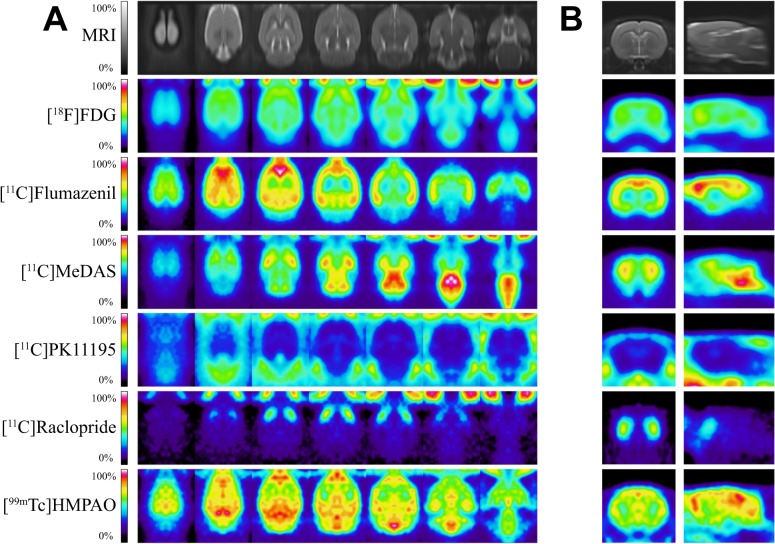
Tracer-specific PET and SPECT templates. (A) Different horizontal brain sections, and (B) sagittal and coronal sections.
